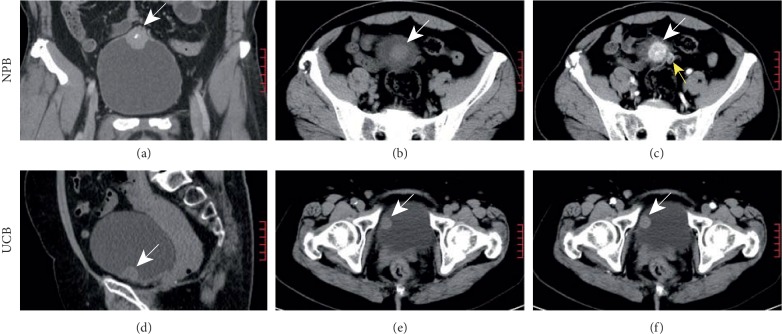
Computed tomography of NPB and UCB. (a–c) A solitary solid NPB with oval profile and intense enhancement, arteries can be observed (yellow arrow). (d–f) A papillary UCB with mild enhancement. NPB = nonfunctional paraganglioma of the bladder; UCB = urothelial carcinoma of the bladder.
